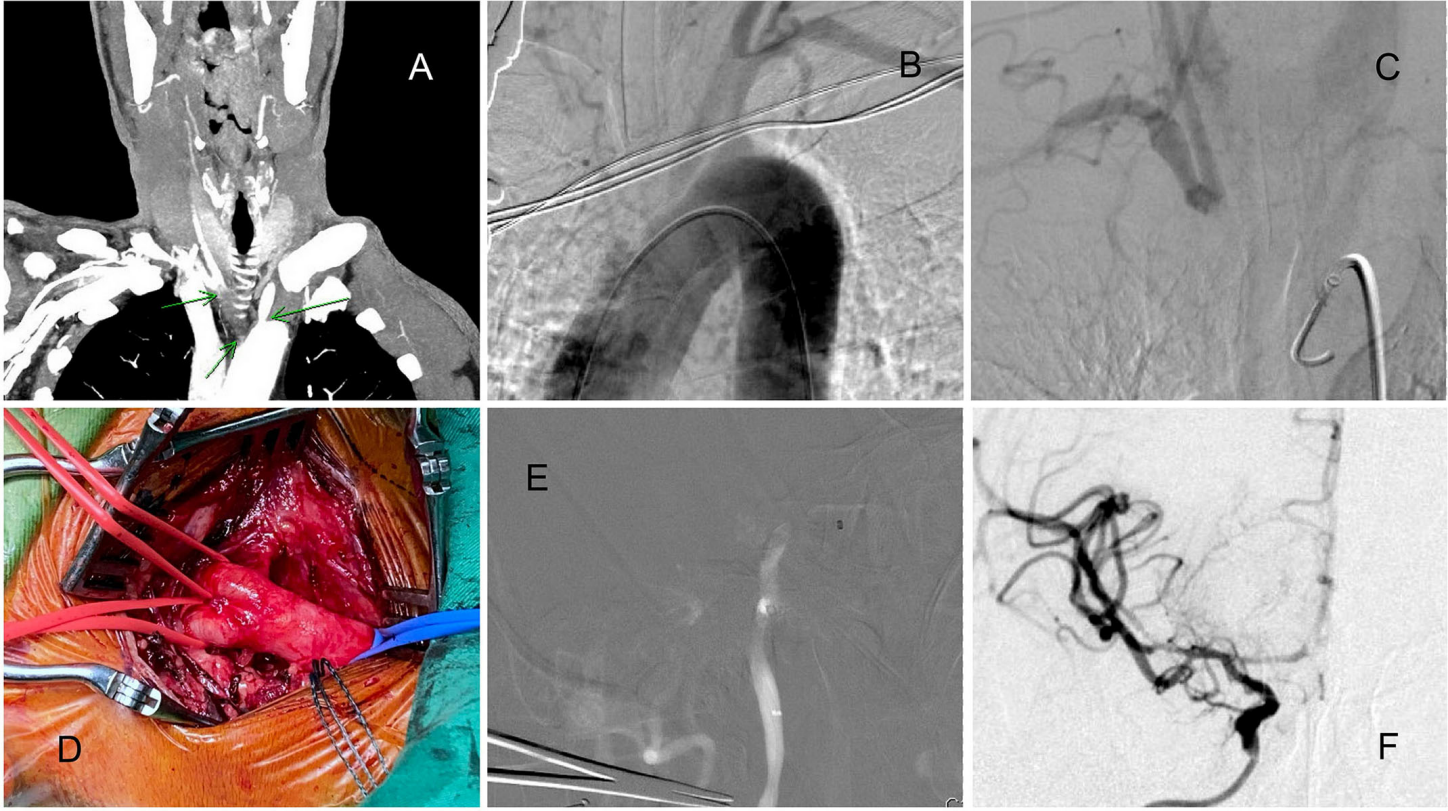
A patient with acute ICA and MCA total occlusion with chronic brachiocephalic trunk occlusion. (A) CTA indicating brachiocephalic trunk occlusion. (B) Aortogram indicating brachiocephalic trunk occlusion. (C) Retrograde collateral flow to the right CCA. (D) The carotid artery was directly explored from the neck. (E) The Sofia aspiration catheter was navigated to the occlusion site. (F) After 2 aspiration passes, total recanalization was achieved.
